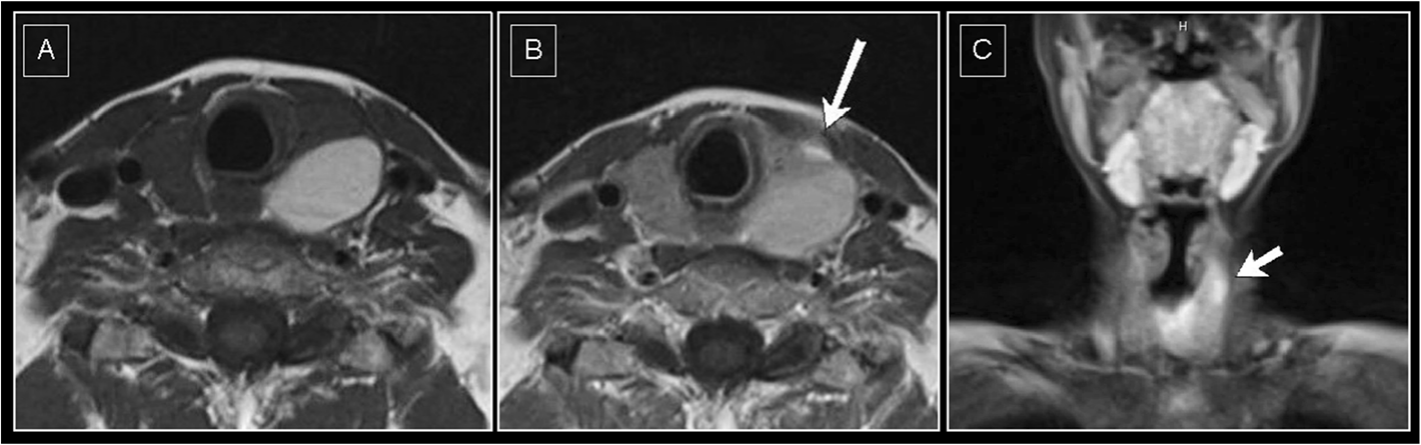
Dynamic contrast agent enhanced MRI: small focal arterial hypervascularization at the ventral part of the capsule of the left-sided parathyroidal cyst. a T2w, axial orientation (b) T2w, axial orientation, the white arrow indicates focal arterial contrast agent enhancement (c) T2w coronal orientation, the white arrow indicates focal arterial contrast agent enhancement
